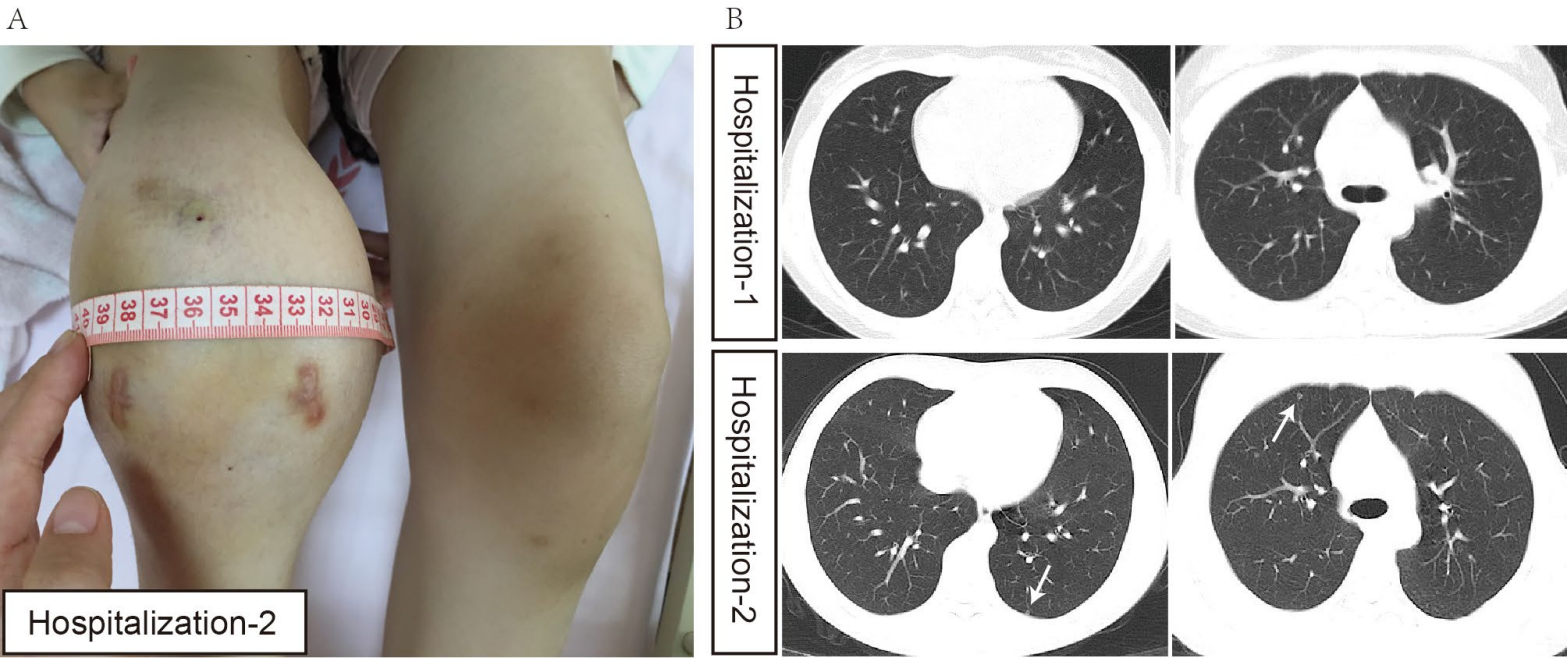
Clinical features of the case. (A) Clinical view of the right knee joint, obviously swollen compared to left knee joint. (B) Computed Tomography (CT) scan showed multiple small nodules (white arrows) in both lungs in second hospitalization
